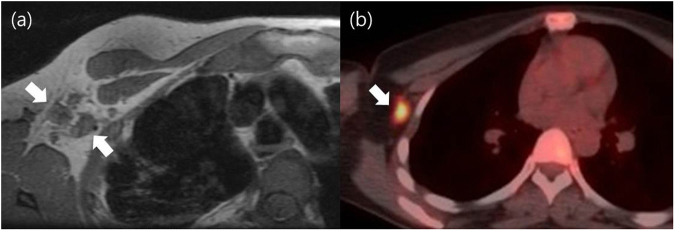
Transverse T1-weighted breast MR images (a) and axial PET/CT images (b) after neoadjuvant chemotherapy in 34-year old woman with invasive ductal carcinoma in her right breast. Breast MR image shows four suspicious nodes (arrows indicate two suspicious nodes among four) and PET/CT image shows FDG uptake (arrows) with a maximum standardized uptake value of 9.2. Axillary lymph node dissection revealed nine lymph node metastases (ypN2).
